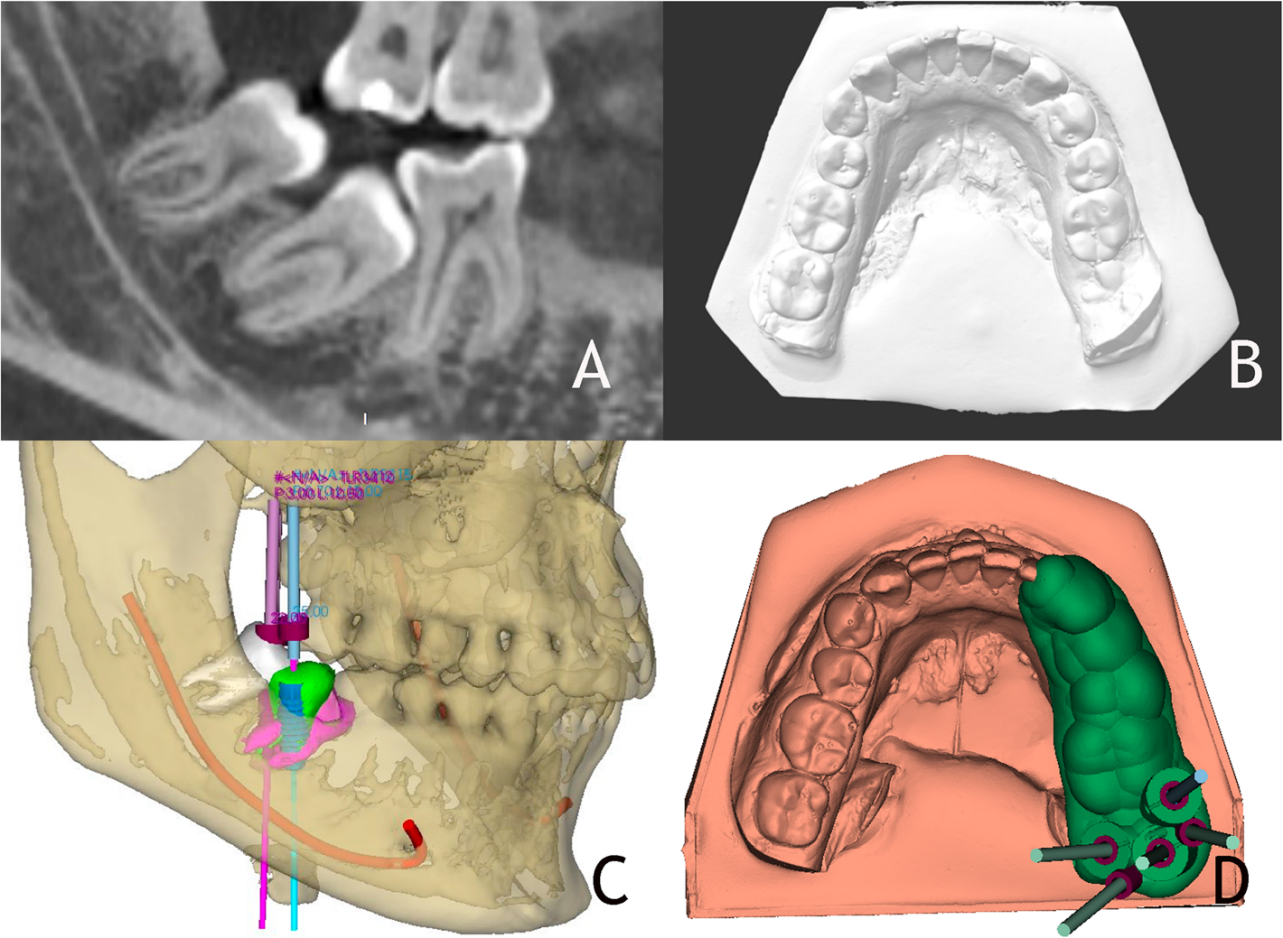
a An 18-year-old male patient was referred with the mandibular third and second molar included. Sagital view in CBCT (White Fox) reveals the possibility of performing an autotransplantation. b Dental models scanned by scanner extraoral (EVO). c Virtual autotransplantation (green tooth) of tooth #48 (white tooth) into the place of tooth #47 (pink tooth), and drilling planning procedure. d Surgical template and models exported as STL files and sent to a 3D printer for fabrication
